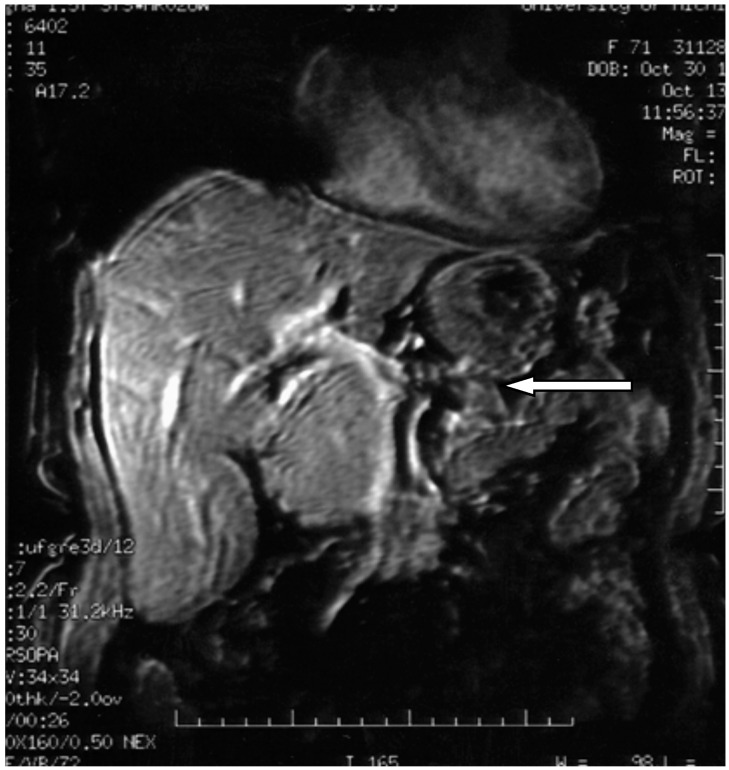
3D axial magnetic resonance imaging showing pancreatic polypeptide-producing tumors in the head of the pancreas (arrow) [18].
